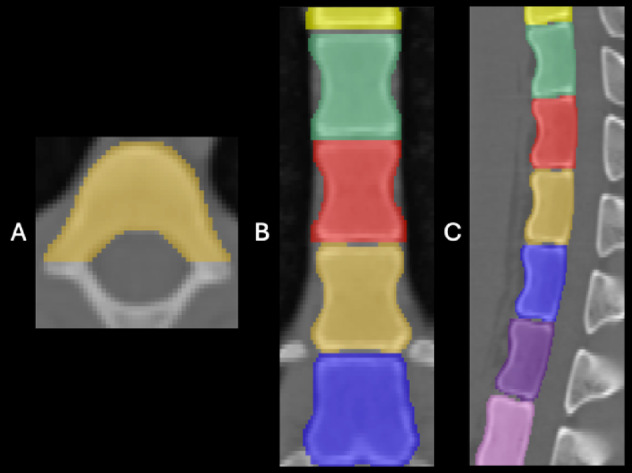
Segmentation of the vertebral bodies of a Göttingen minipig in a non-contrast CT examination with a radiation dose of 10.01 mGy in A axial, B coronal and C sagittal view. Th9 is annotated in brown. mGy = milligray.
